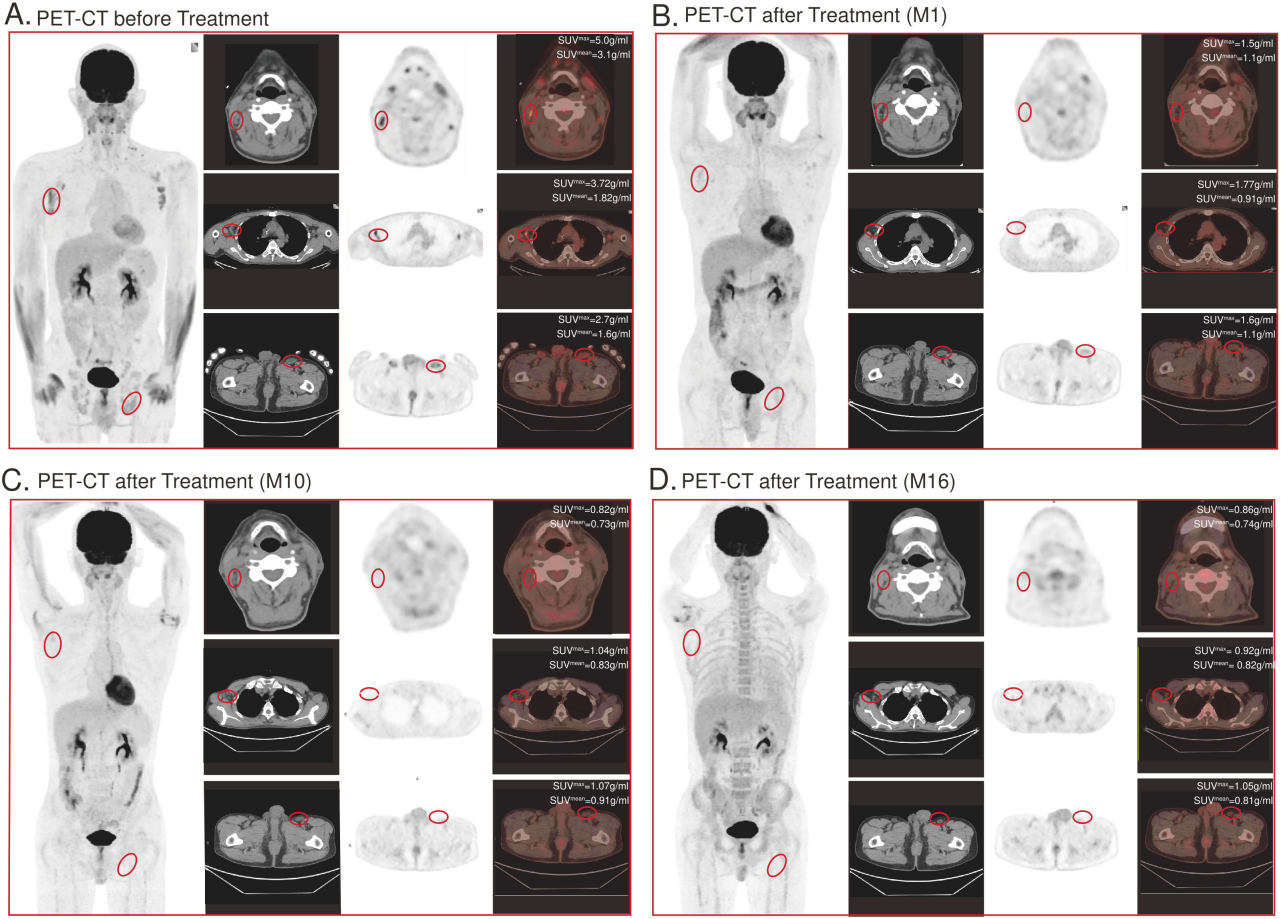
CD7-RTX CAR T cell treatment significantly reduces tumor burden. Top panel shows CT images, the middle panel shows the radionuclide distribution, and the bottom panel shows PET-CT fusion images. (A) PET-CT image demonstrates lesions of high uptake prior to treatment: (1) over the right temple (View 1), (2) right axilla (View 2), and (3) left inguinal region (View 3). (B–D) PET-CT image 1 month, 10 months, 16 months post-treatment. Absence of bulk disease appreciated: (1) over the right temple (View 1), (2) right axilla (View 2), and (3) left inguinal region (View 3).
